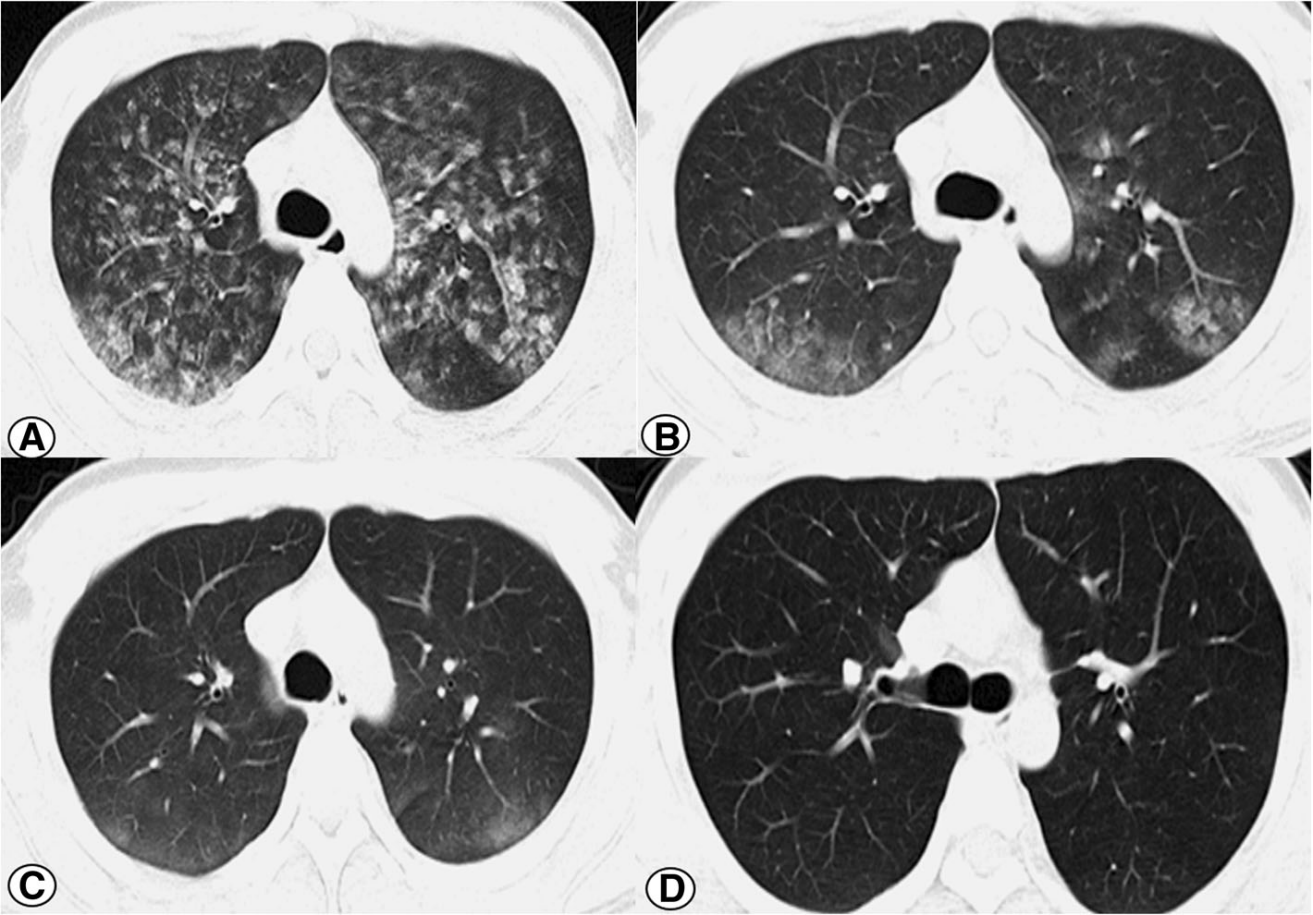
a-d Chest computed tomographic scan obtained 2 h, 25 h, 3 days and weeks after the surgery. It was shown that bilateral pleural effusion gradually decreased over time and completely disappeared on follow up
